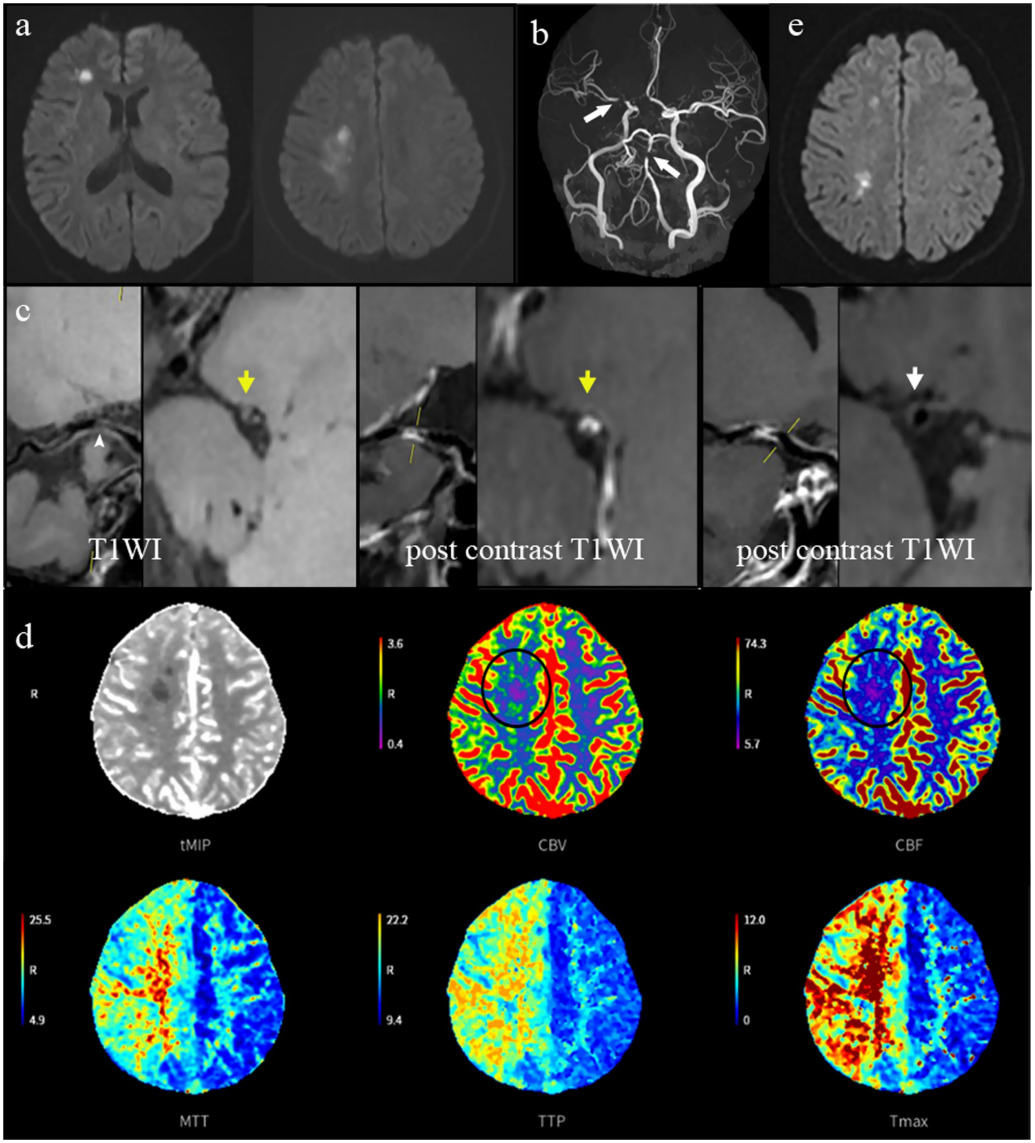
This case represents a patient with recurrent stroke. A 52-year-old male with a history of BZI experienced a recurrent stroke event 2 months after aggressive medical therapy. Baseline DWI (a) revealed BZI, with external (cortical) lesions located in the territories supplied by the anterior and middle cerebral arteries, as well as internal (subcortical) lesions adjacent to the lateral ventricles within the centrum semiovale. TOF-MRA (b) shows severe stenosis in the M1 segment and basilar artery. HRVWI (c) curve planes and cross-sections show the culprit lesion (yellow arrow) in the M1 segment, which was characterized by intraplaque hemorrhage (white arrowhead) on T1WI, marked contrast enhancement, and a negative remodeling pattern when compared to the reference site (white arrow). The remodel index was measured as 0.57. CTP (d) shows unfavorable collateral status, evidenced by reduced CBF and CBV (circle), along with prolonged MTT, TTP, and Tmax. DWI during follow-up (e) reveals NICLs, presenting as rose-shaped multiple inner border zone infarcts along the center of the ipsilateral centrum semiovale. BZI, border zone infarction; DWI, diffusion-weighted imaging; HRVWI, high-resolution vessel wall imaging; T1WI, T1-weighted imaging; CTP, computed tomography perfusion; CBF, cerebral blood flow; CBV, cerebral blood volume; MTT, mean transit time; TTP, time to peak; Tmax, time to maximum of the residue function; NICLs, new ischemic cerebral lesions.
